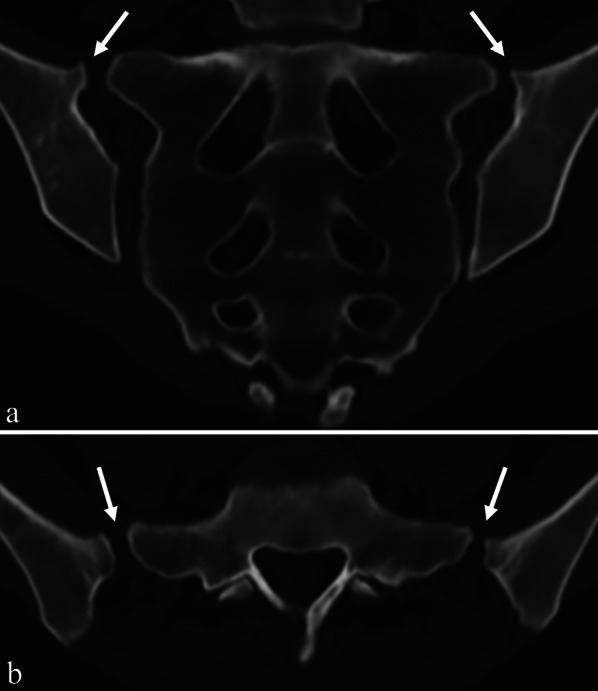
New sacroiliac joint (SIJ) variant with extra joint facet superiorly. Semi-coronal (a) and semi-axial (b) synthetic CT images depict a newly found SIJ variant in a 27-year-old women, consisting of an accessory joint cranial of the cartilaginous part of the SIJ, between bony projections from the ilium (arrows) and the posterosuperior edge of S1 vertebra
